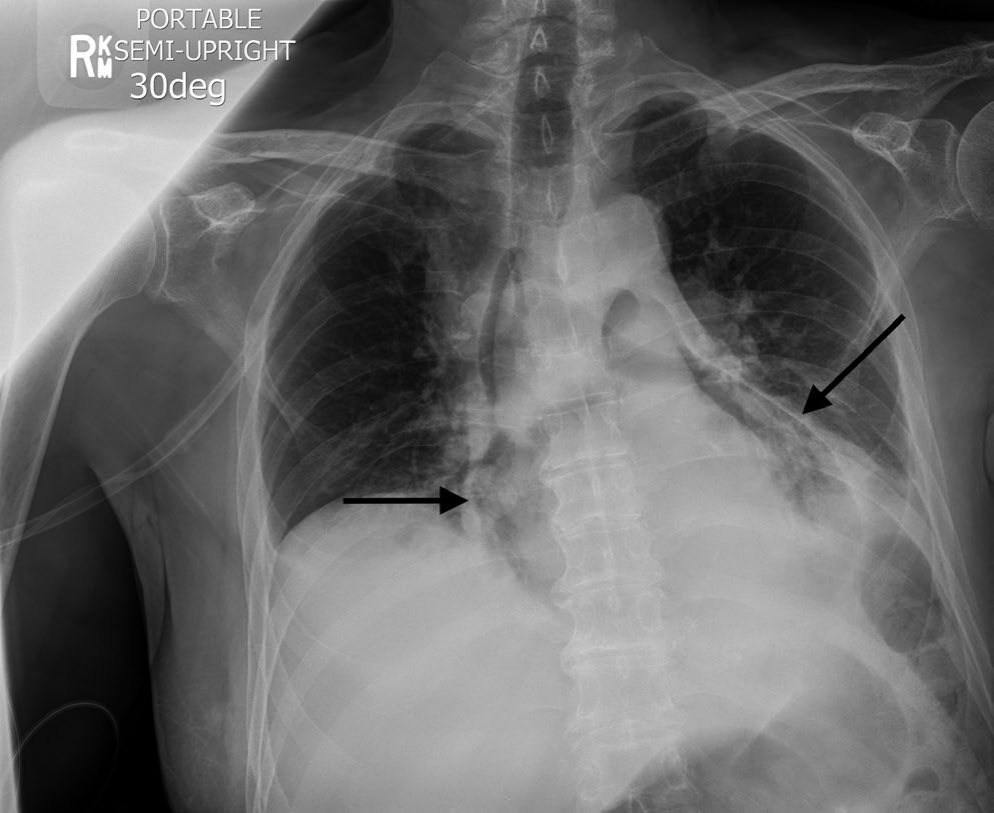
Chest radiograph which shows a band of gas curving around the cardiac chambers, with a sharp demarcation by the pericardium (black arrows)
